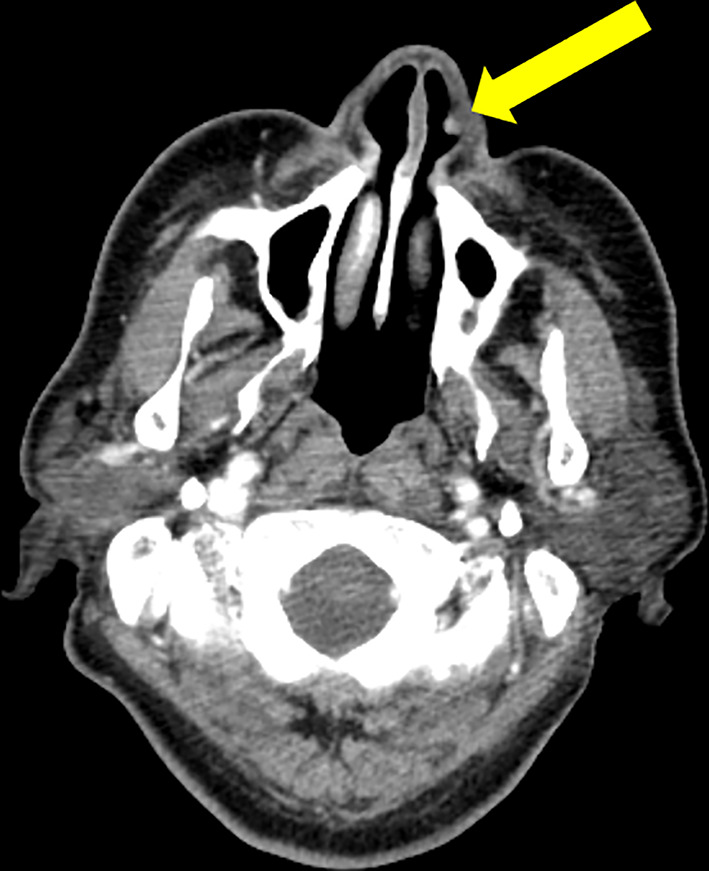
Axial enhanced computed tomography scan images of nasal vestibule show small elevated tumor (yellow arrow).
